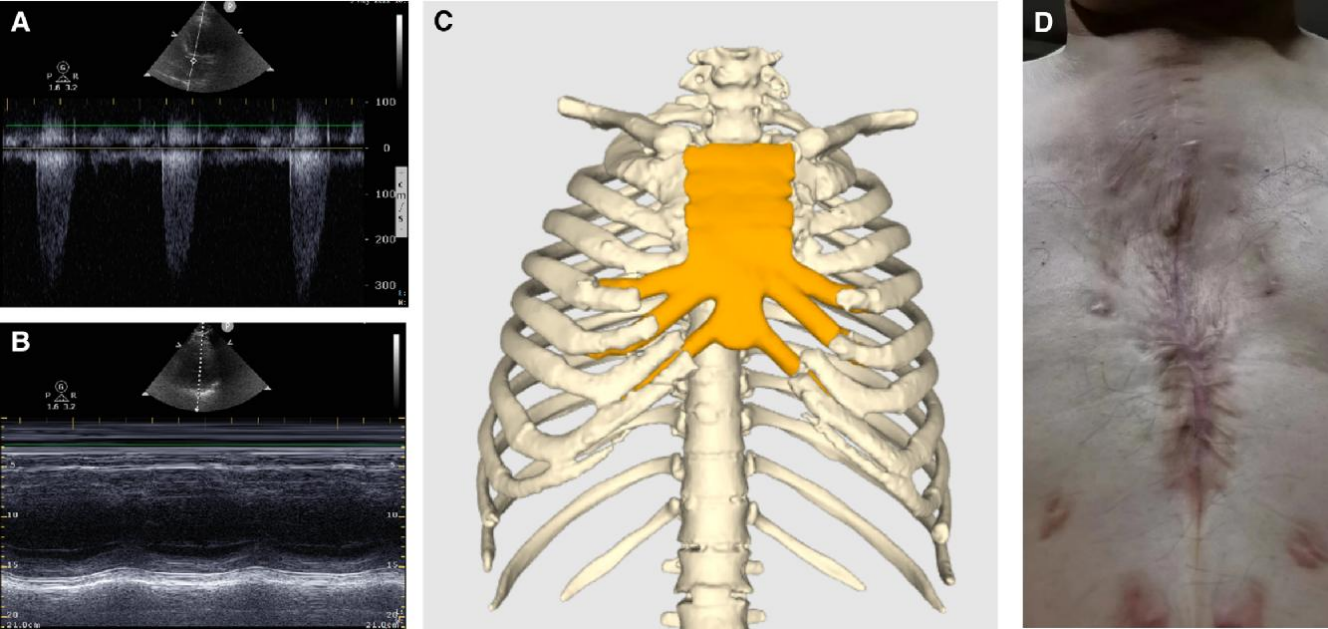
Transthoracic echocardiography revealed that bioprosthetic aortic valve worked properly without perivalvular leakage (*A*), and left ventricular systolic function was improved (M-mode echocardiography: ejection fraction 52.0%; *B*). 3D reconstruction image of the chest demonstrated that the location of the polyether ether ketone implant remains stable (*C*). The gross observation of chest wall structures is shown in *panel D*.
